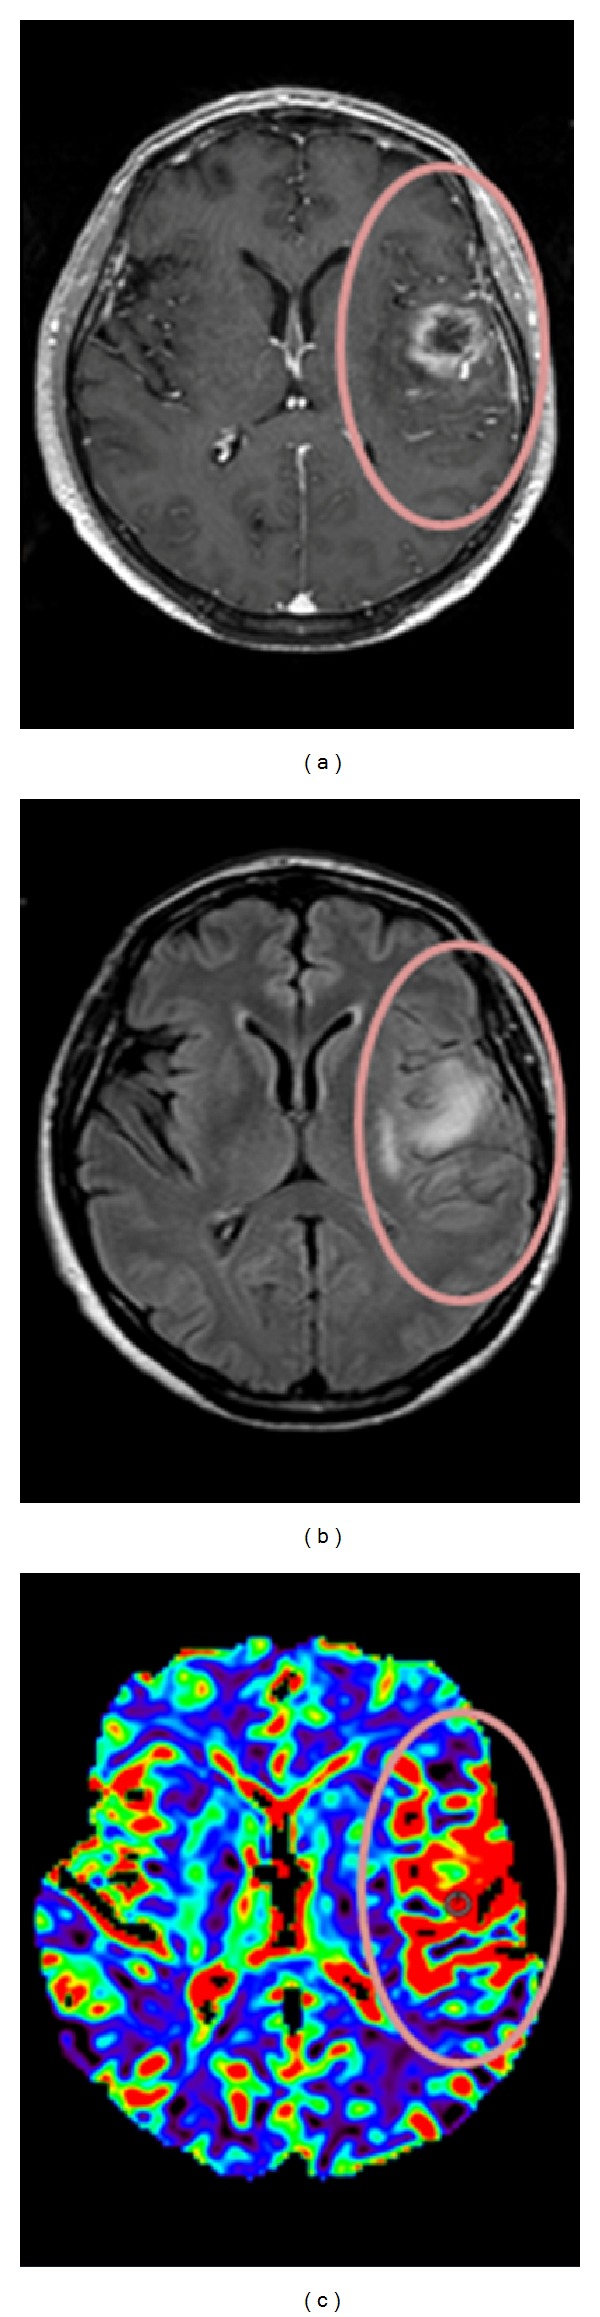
Axial coregistered contrast-enhanced axial T1-weighted image (a), FLAIR image (b), and CBV map (c) from a patient affected by glioma grade IV. In the CBV map (c) warmer colors indicate higher CBV values suggesting higher perfusion and neovascularization. Comparison of CBV map (c) and contrast-enhanced axial T1-weighted image highlights a mismatch area (surrounded by the circle) corresponding to the extension of the high perfusion area outside the contrast-enhancement: this indicates a more extensive neovascularization than that shown by conventional MRI (a, b).
